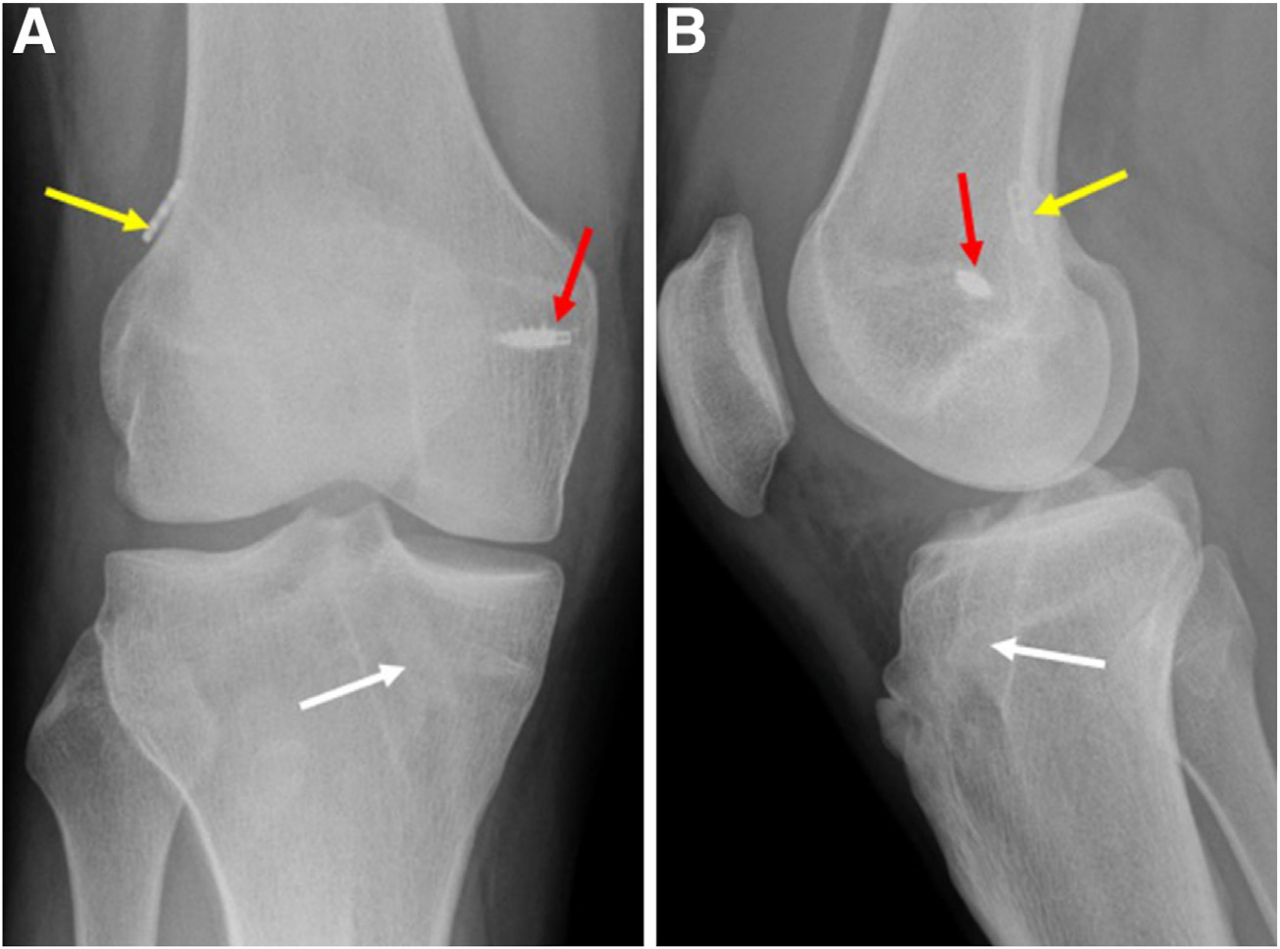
(A and B) Anteroposterior and lateral right knee preoperative radiographs showing previous ACL tunnel position in the tibia (white arrow), femur button position (yellow arrow), and previous MCL repair screw position (red arrow). (ACL, anterior cruciate ligament; MCL, medial collateral ligament)
